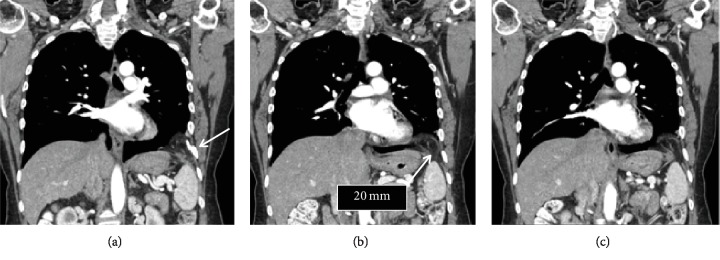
Coronal CT scan images showing diaphragmatic omental herniation (right side of each image). The arrow in (a) shows a healed rib fracture. The arrow in (b) indicates the hernia, which can be seen in each image.
